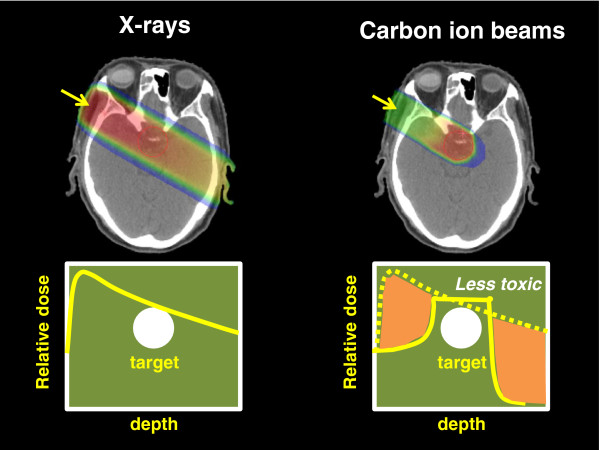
The difference of dose distribution by one port between carbon ion beams and X-rays.
